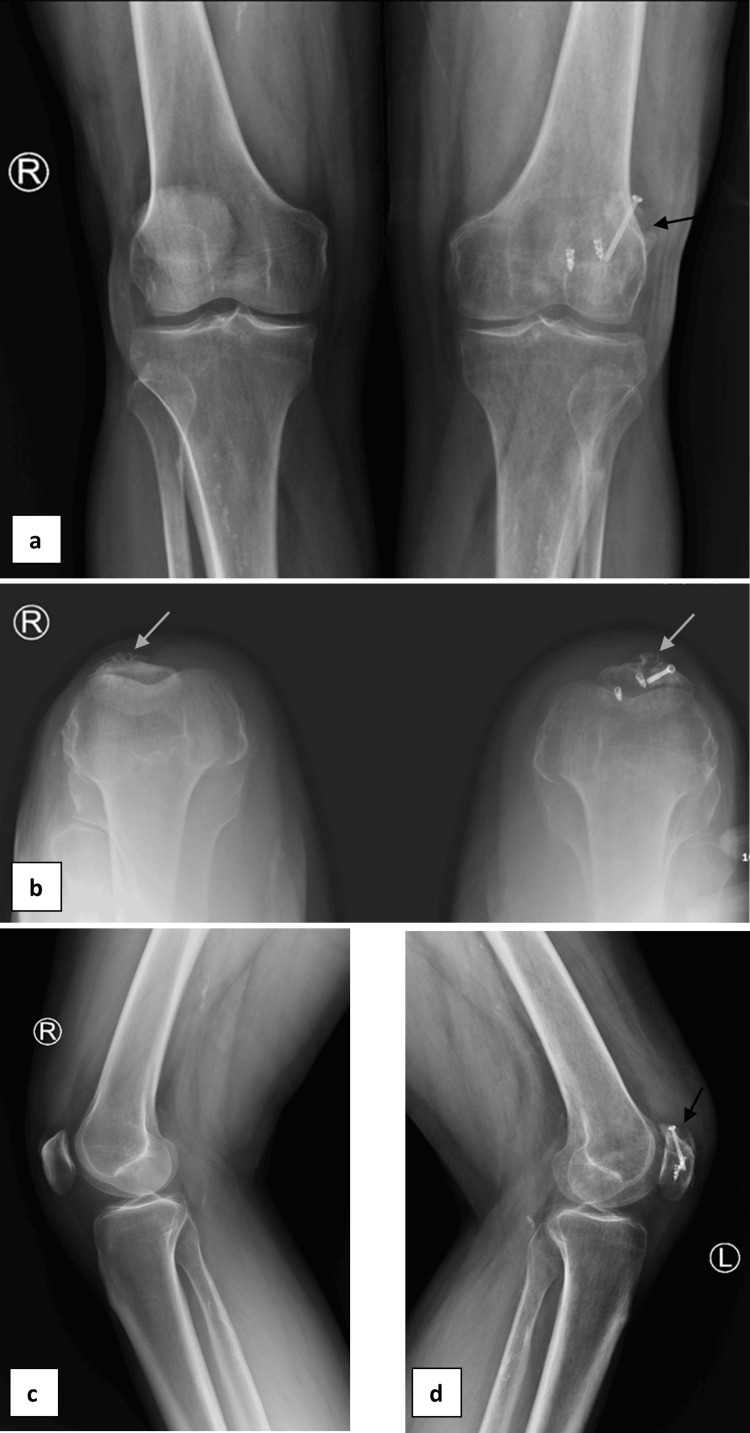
Follow-up radiographs of both knees (a) Standing AP view, (b) skyline view, showing osteophytes along the anterior cortex of the patella, more pronounced on the left side (grey arrows), (c) right lateral view, and (d) left lateral view. The black arrows indicate the stable position of the fixation.
